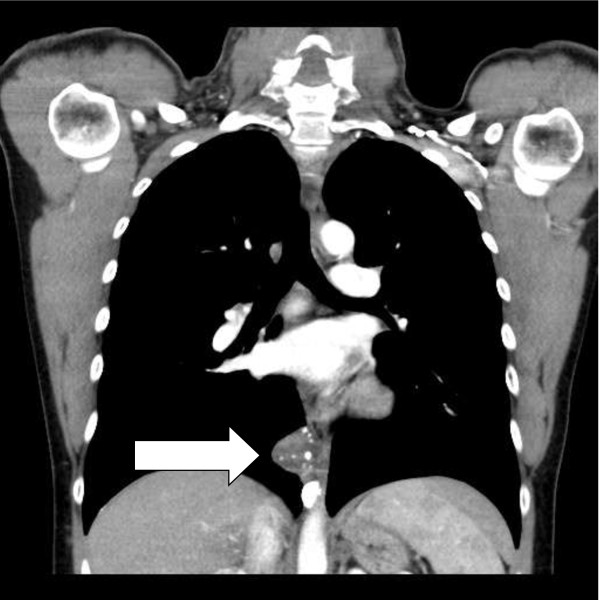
CT image of CFT in the paraspinal region (arrow).
